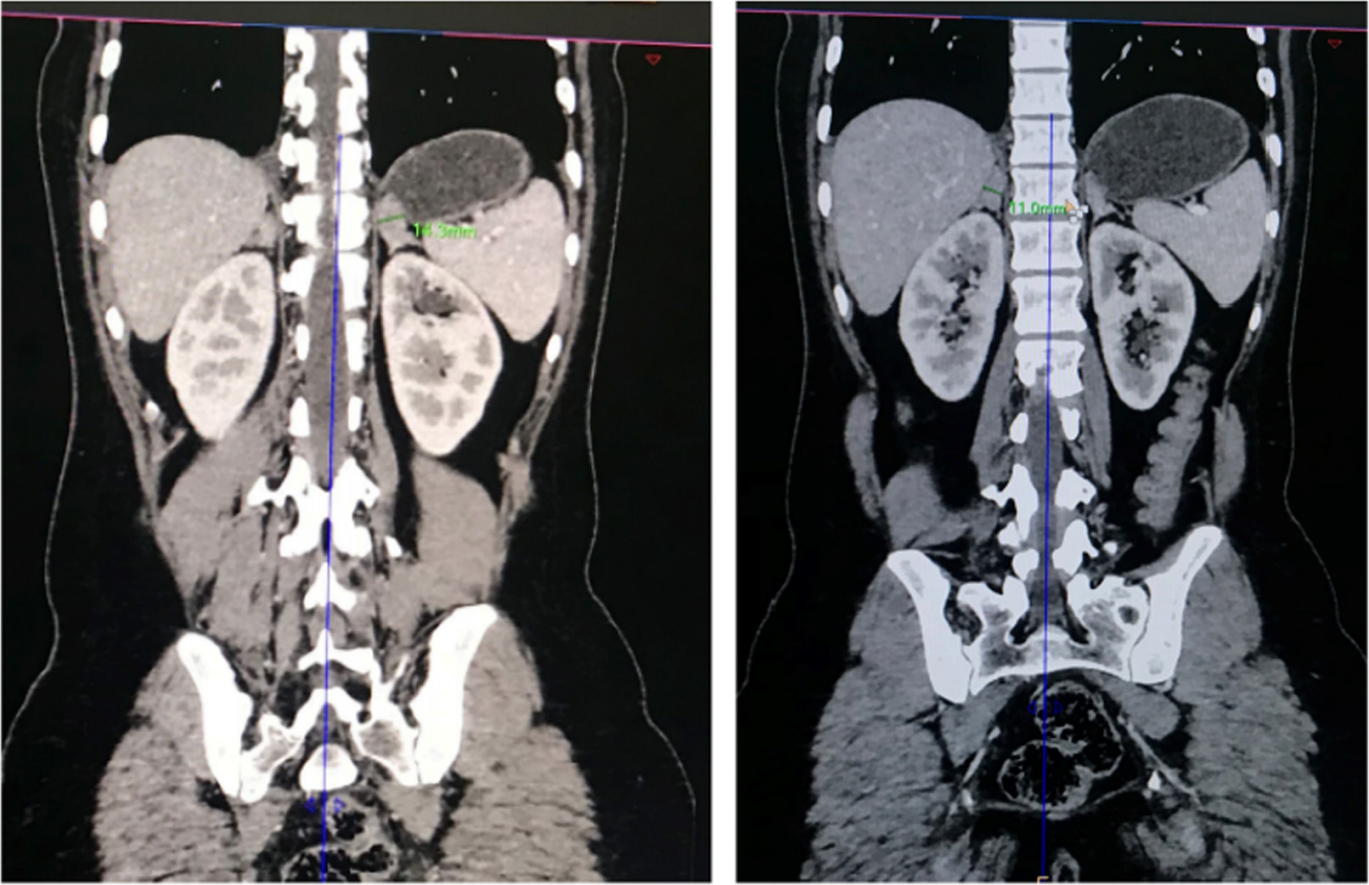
Coronal CT image showing bulky body of left and right adrenal glands measuring 14.3 mm and 11.0 mm, respectively
